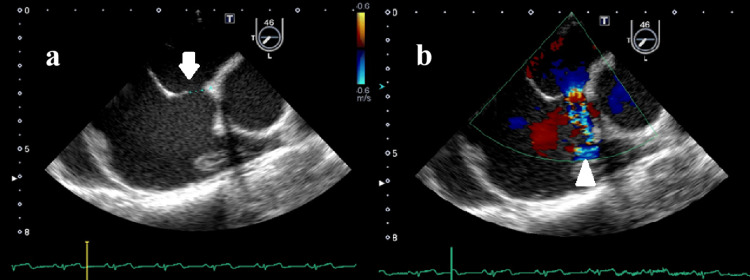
Transeshophageal echocardiography shows (a) atrium septal defect and (b) jet flow from left atrium to right atrium
